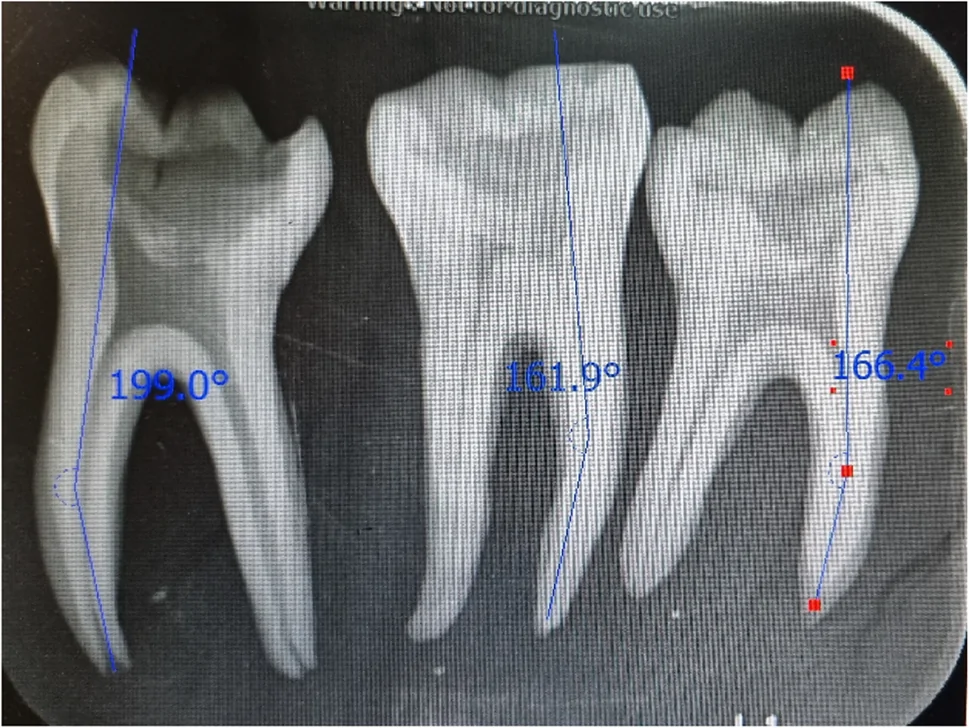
Periapical radiograph demonstrating root curvature determination of the samples
